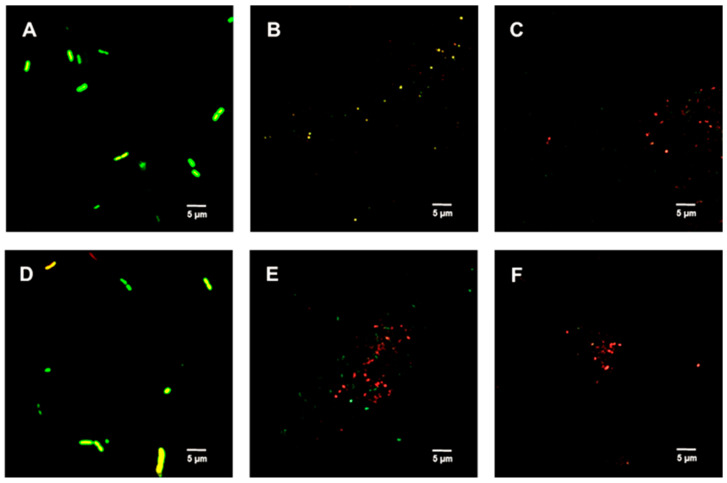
Live/Dead assay of *Bacillus* spp. spores with TFDG at 60-min incubation. The green indicates viable spores, while the red indicates non-viable spores. (A) *B. cereus* spores control; (B) *B. cereus* spores with 312.5 µg/mL TFDG; (C*) B. cereus* spores with 625 µg/mL TFDG; (D) *B. subtilis* spores control; (E) *B. subtilis* spores with 312.5 µg/mL TFDG; and (F) *B. subtilis* spores with 625 µg/mL TFDG.
